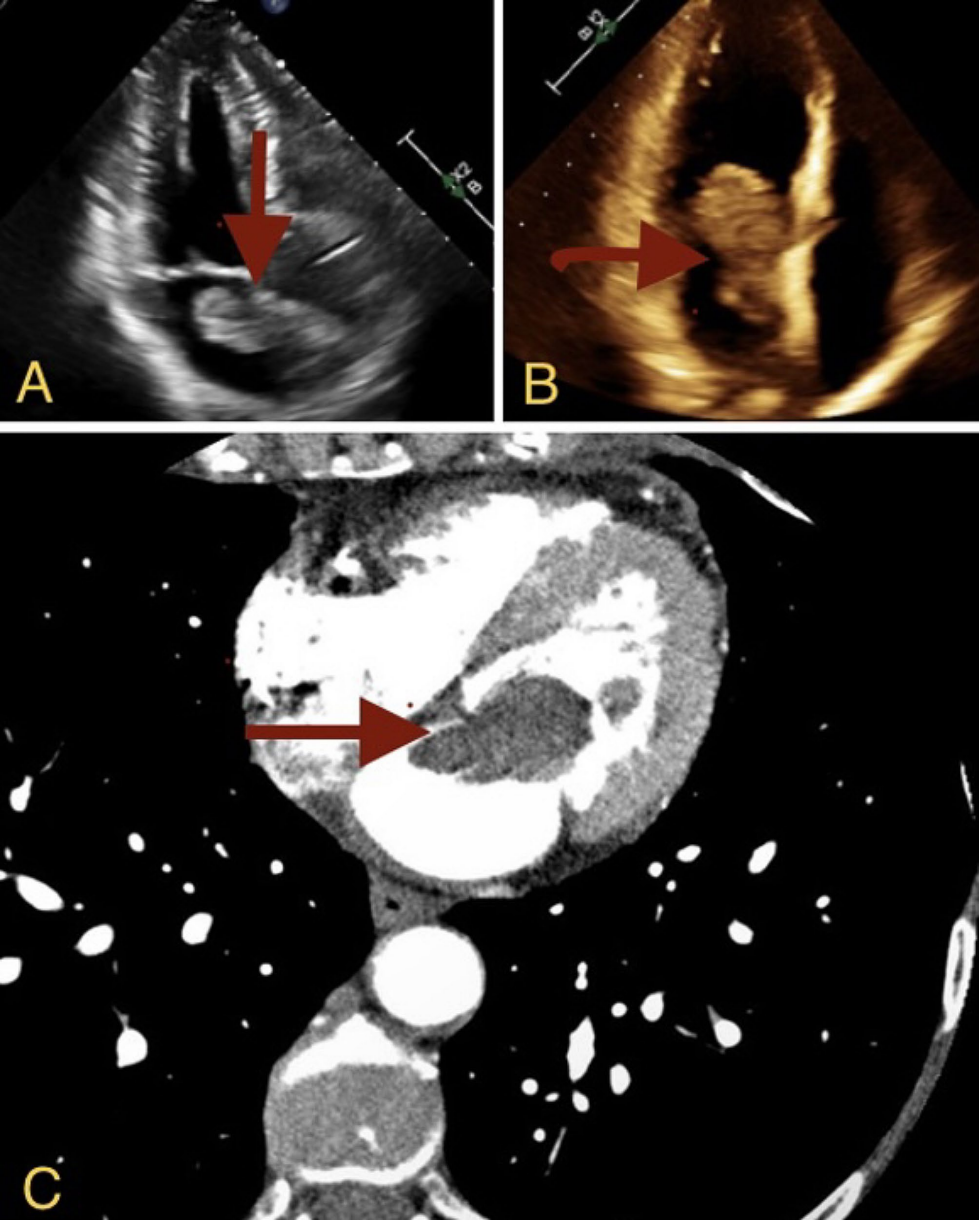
Transthoracic echocardiogram (TTE) displaying the extent of hemodynamic impact from the left atrial mass. (A) The mass was initially contained in the left atrium (apical 3-chamber view). (B) Follow-up imaging showing prolapse of the mass through the mitral valve into the left ventricle (apical 2-chamber view). (C) Computed Tomography (CT) (axial slice through the mitral valve) with contrast also showing the extent of left ventricular obstruction with high risk of rupturing and embolizing with possible catastrophic complications
